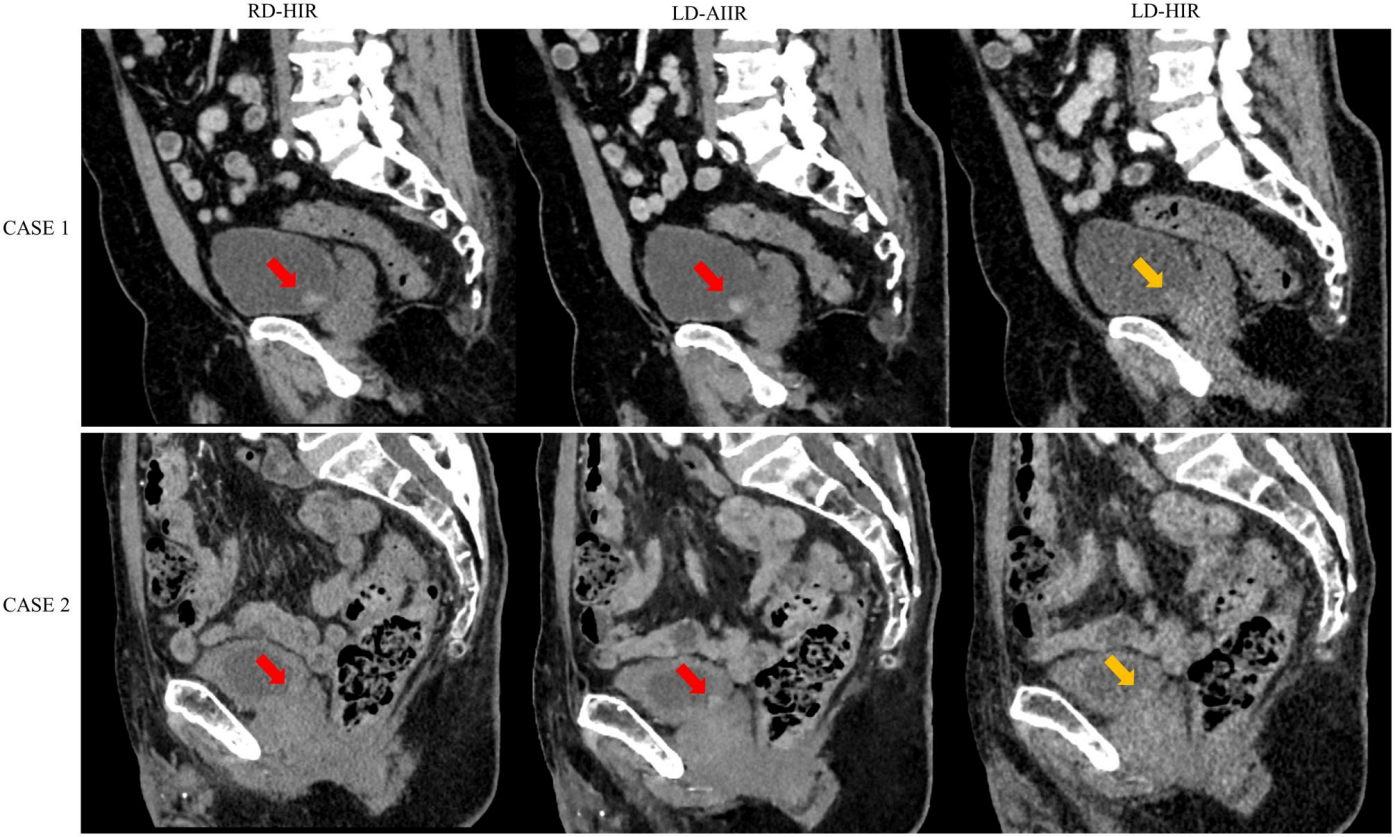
Corticomedullary phase CT images of a 75-year-old man (CASE 1) and a 87-year-old man (CASE 2) at routine-dose with hybrid iterative reconstruction (RD-HIR), low-dose with artificial intelligence iterative reconstruction (LD-AIIR), and with hybrid iterative reconstruction (LD-HIR). Pathology results confirmed both cases were positive for bladder cancer (arrow). The diagnostic confidence on RD-HIR, LD-AIIR, and LD-HIR was graded 4, 4 and 3 for CASE 1; and 4, 4, and 2 for CASE 2, respectively, by both radiologists.
